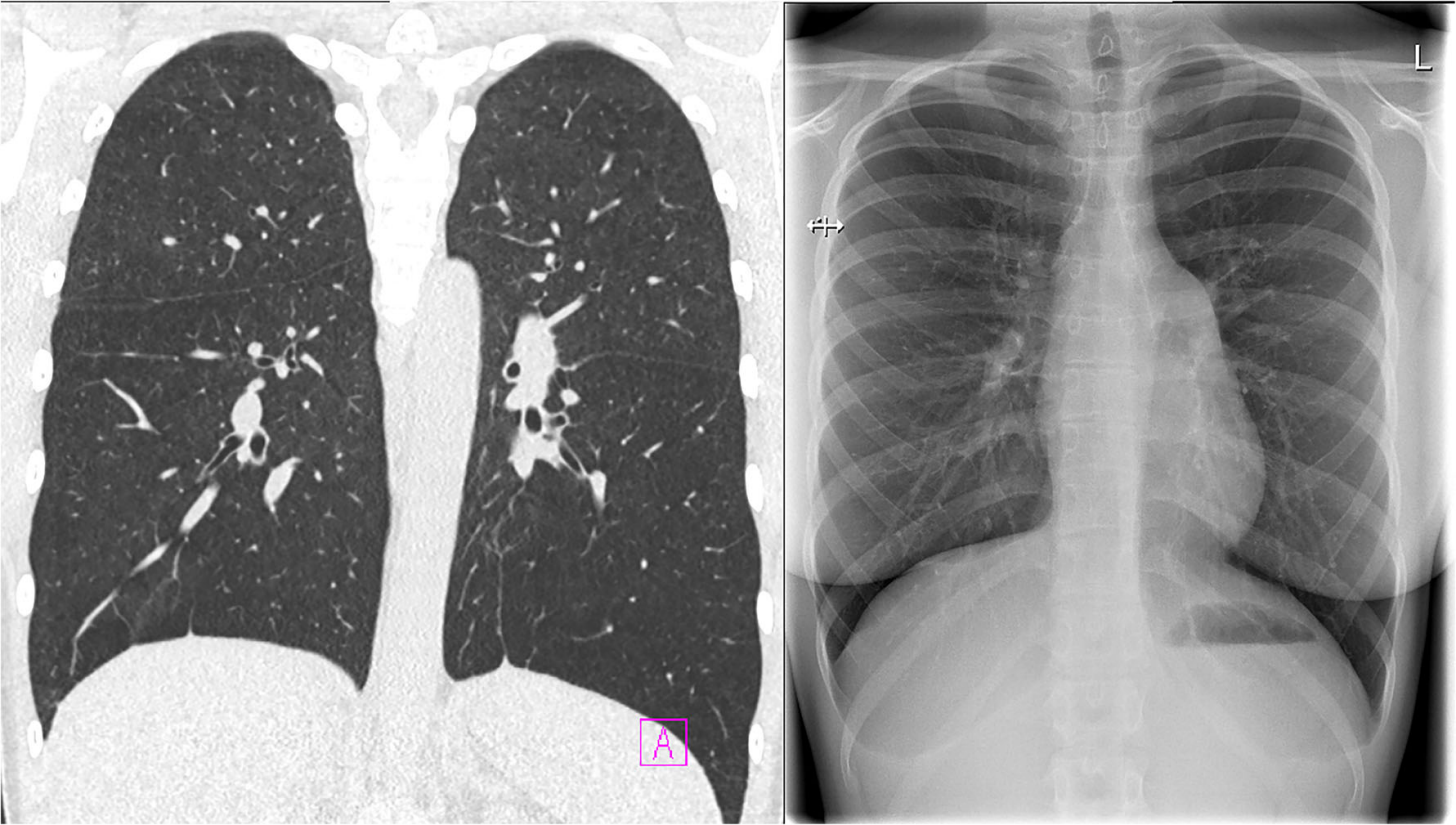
Contrast enhanced coronal chest CT (left) and X‐ray (right) images with panlobular emphysema in patient 1–1
